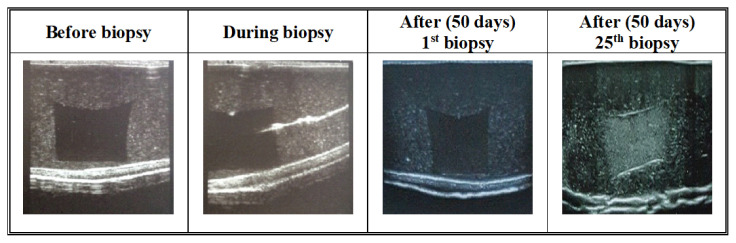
Ultrasonographic images of SH-P before, during and after first and fourth biopsy.
